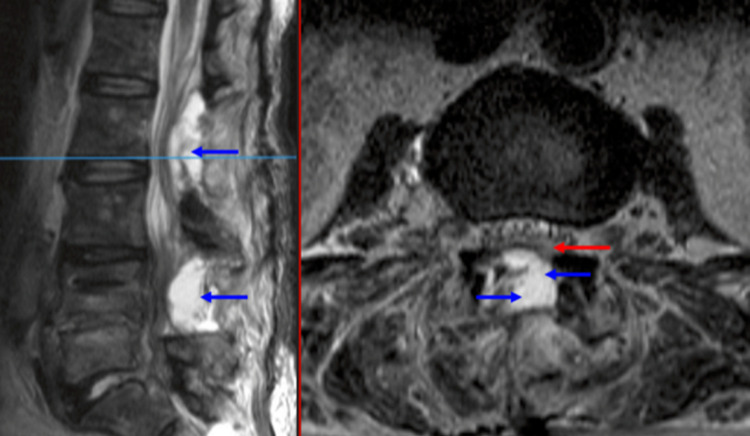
Post-operative T2 TSE Sagittal and Axial MRI showing posterior fluid collections in keeping with recent surgery (blue arrows) and reduction in subdural collection (red arrows). TSE - Turbo Spin Echo; MRI - Magnetic Resonance Imaging
